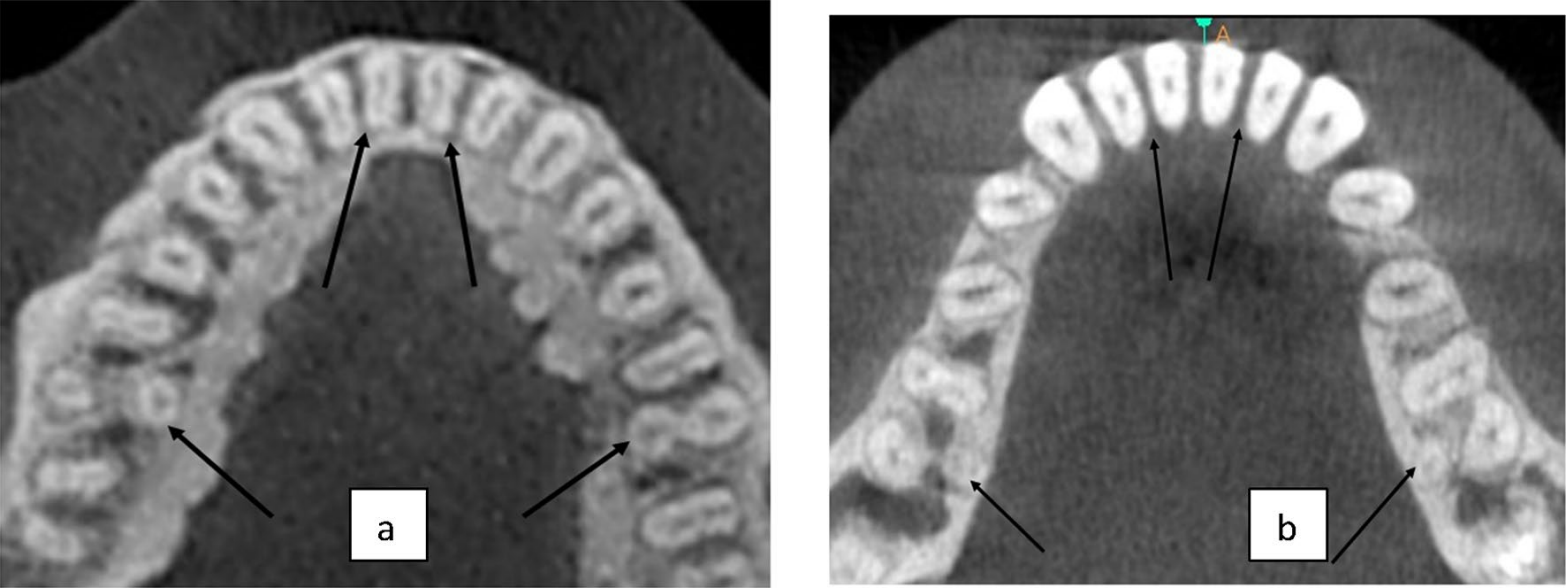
Axial section of CBCT scans showing a) Complicated and b) Simple root canal configuration of Mandibular Incisors in presence of DLR in MFMs.
